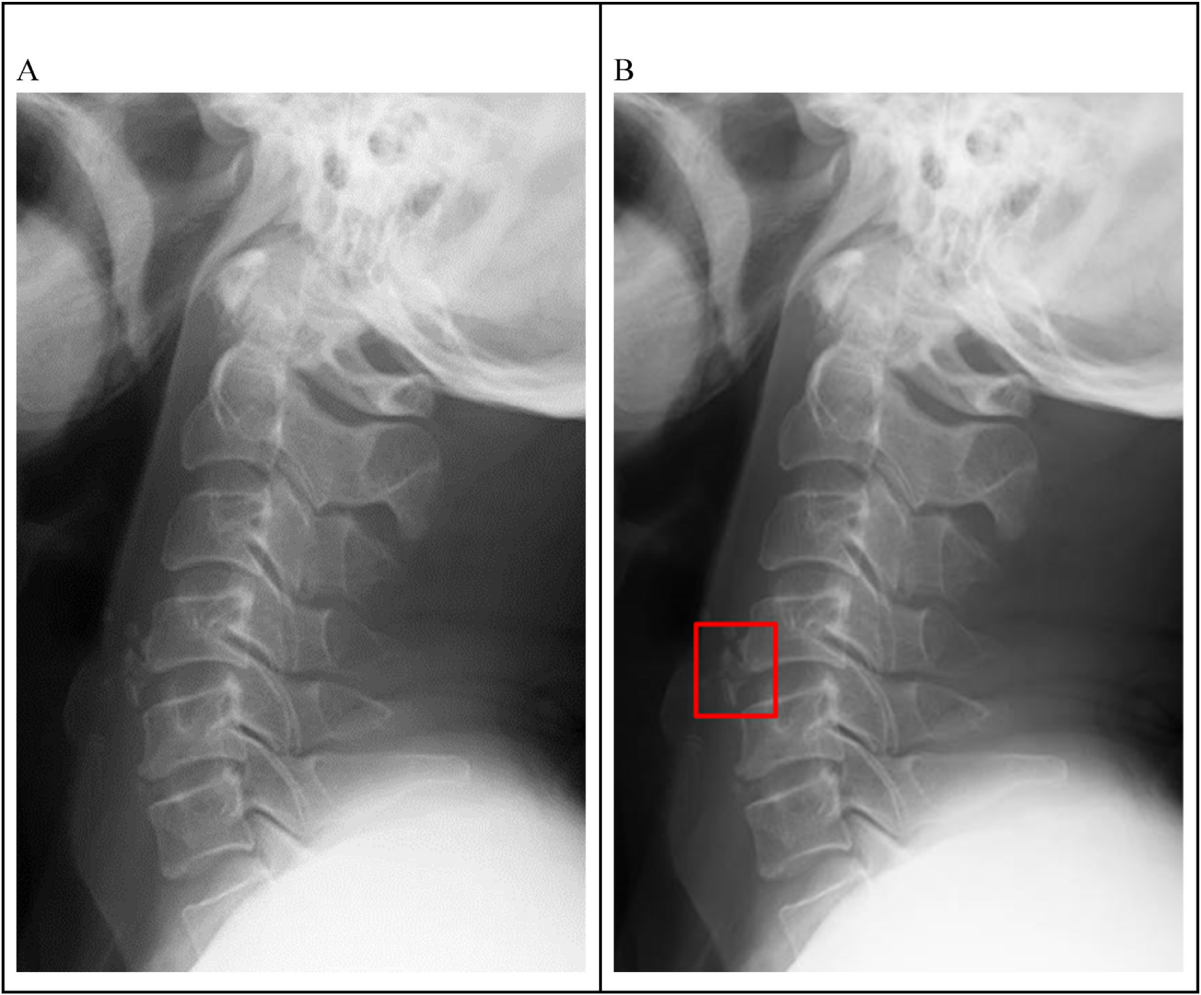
Lateral cervical radiograph with a CT report of C4/5 osteophyte fracture. The fracture is visible on the radiograph and was labeled accordingly.
